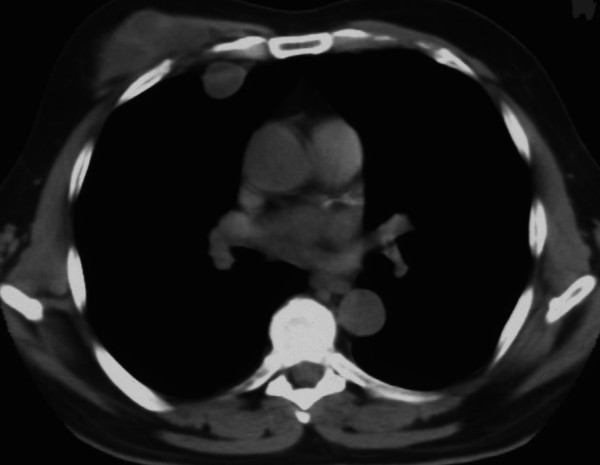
CT axial scans of the thorax showing an intramuscular hypodense tumor with peripheral enhancement which adheres to the body of the right 4^th ^rib. Smaller soft tissue attenuation tumor diameter 2 cm
